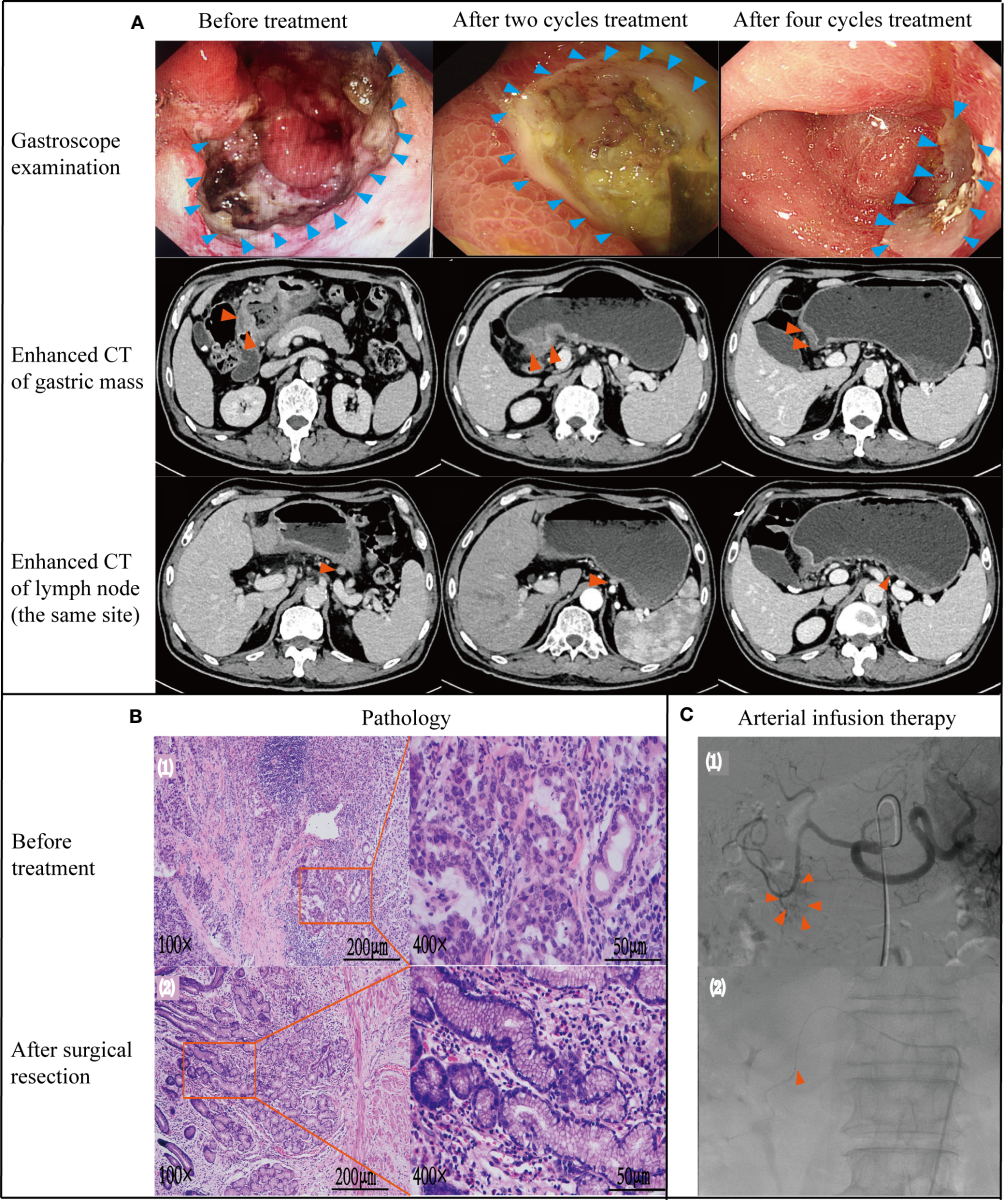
Case data of patient 1. (A) Gastroscope and imaging examination.Changes of tumor or lymph node in gastroscopy and enhanced CT before treatment, after 2 and 4 cycles treatment. (B) Pathology examination (1): biopsy pathology before treatment (adenocarcinoma);(2): pathology after surgical resection (no tumor cells found) (C) Treatment process under DSA (1): angiography showed that the feeding artery of the tumor was gastroduodenal artery; (2): microcatheter tip placement location (in gastroduodenal artery).
